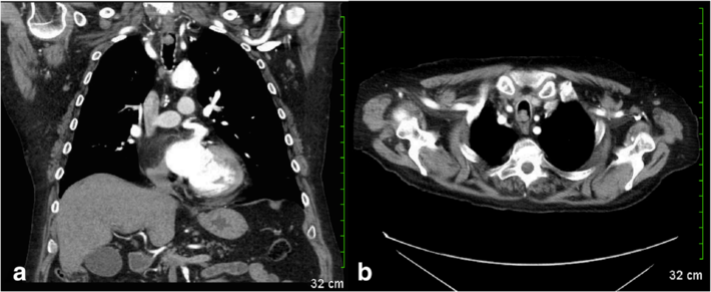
a (left): Chest computed tomography scan sagittal view demonstrating a 1.5 cm nodular soft tissue lesion within the upper subglottic thoracic trachea, 8 cm from carina. b (right): Axial view of the lesion resulting in greater than fifty-percent reduction in cross-sectional area of the tracheal lumen
